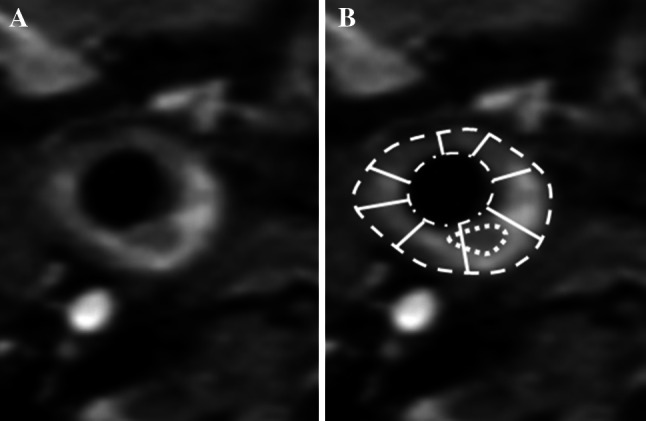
A T1-weighted contrast-enhanced fat saturation image through the common carotid depicts the vessel morphology including the lumen area (*dot-dash line*), total vessel area (*dashed line*) and mean wall thickness (a value obtained by averaging a number of cords, represented by the solid lines). The wall area is calculated by subtracting the lumen area from the total vessel area. The lipid-rich necrotic core component is also outlined (*dotted line*)
